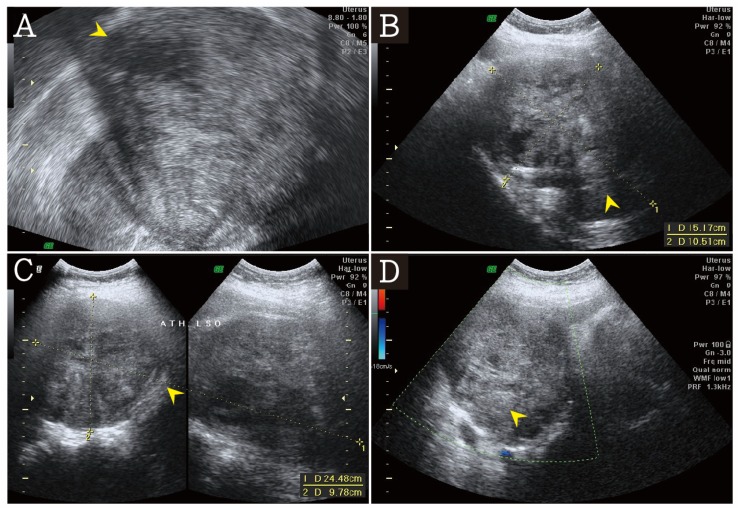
(A) The transvaginal ultrasound revealed a huge heterogeneous echogenicity mass (arrow head). (B,C) The heterogeneous mass was about 15.17 × 24.48 × 10.51 cm^3^ (arrow head). (D) In doppler color flow mapping, no significant blood flow was noted in the heterogeneous mass (arrow head).
